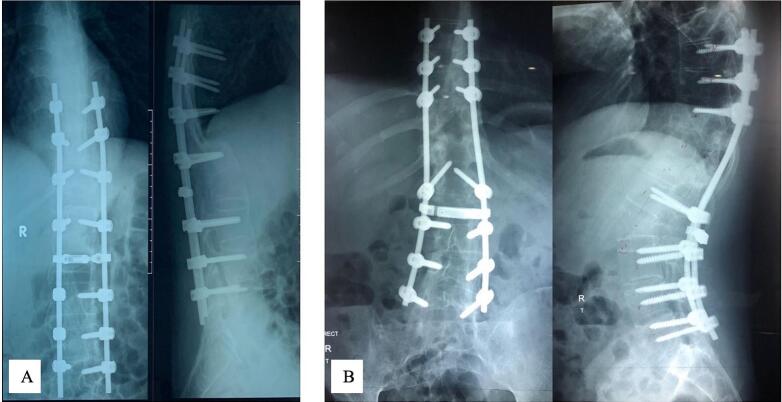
Postoperative radiological examination of thoracolumbar vertebra X-ray in AP and lateral view: (A) case 1; (B) case 2.
